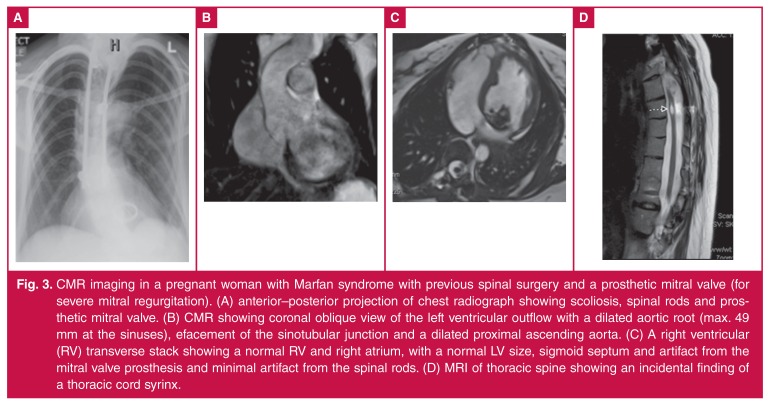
CMR imaging in a pregnant woman with Marfan syndrome with previous spinal surgery and a prosthetic mitral valve (for severe mitral regurgitation). (A) anterior–posterior projection of chest radiograph showing scoliosis, spinal rods and prosthetic mitral valve. (B) CMR showing coronal oblique view of the left ventricular outflow with a dilated aortic root (max. 49 mm at the sinuses), efacement of the sinotubular junction and a dilated proximal ascending aorta. (C) A right ventricular (RV) transverse stack showing a normal RV and right atrium, with a normal LV size, sigmoid septum and artifact from the mitral valve prosthesis and minimal artifact from the spinal rods. (D) MRI of thoracic spine showing an incidental finding of a thoracic cord syrinx.
